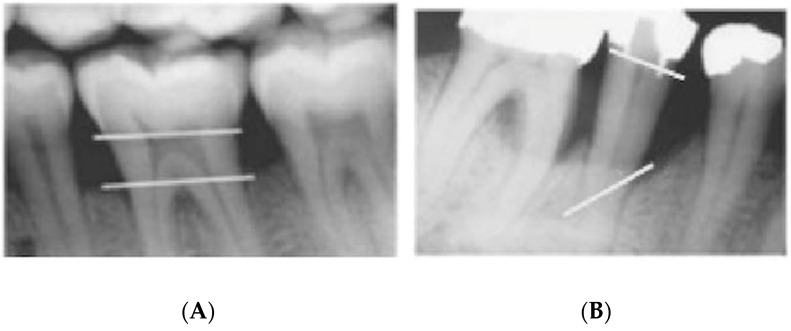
Radiographic interpretation in terms of periodontal bone loss pattern which is classified into (A) horizontal bone loss and (B) vertical bone loss.
